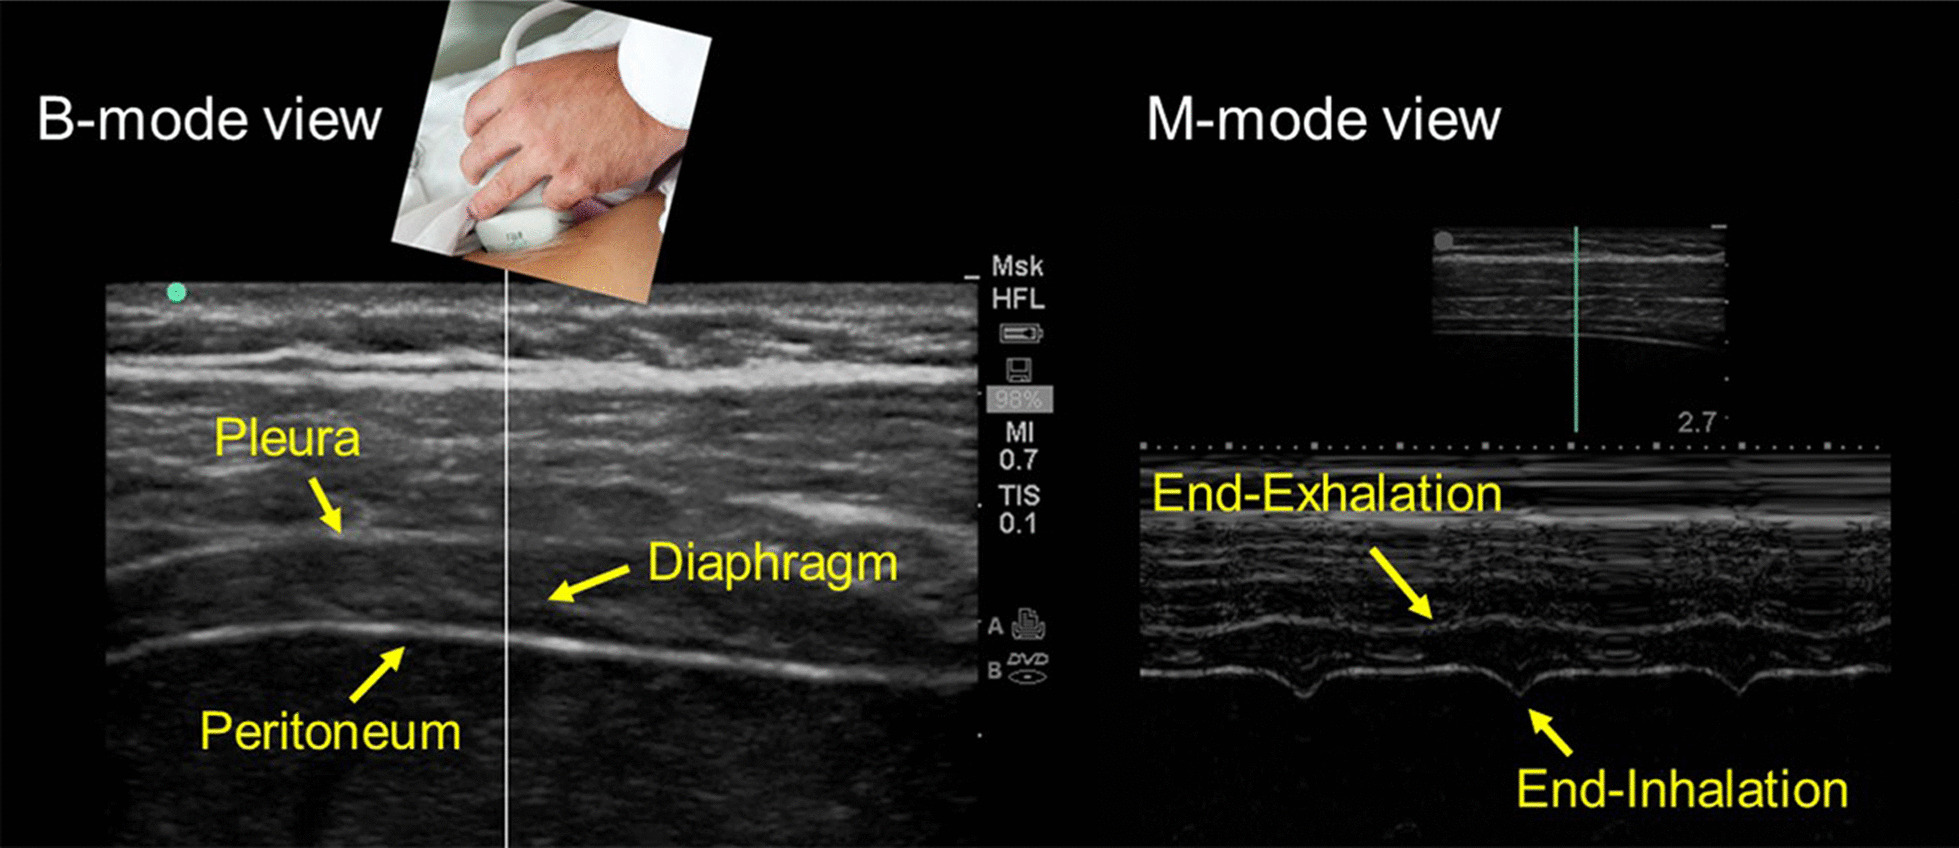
Ultrasound image of the zone of apposition of the diaphragm. In brightness-mode (B-mode; *left panel*), the diaphragm appears as a three-layer structure. In motion-mode (M-mode; *right panel*), the diaphragm is thinnest at end-exhalation and thickest at end-inhalation; stronger diaphragmatic inspiratory efforts are associated with greater tidal thickening of the diaphragm. Reproduced with permission from The American Association for Respiratory Care: Shaikh et al. Respir Care 2019;64:1600–2. The entirety of both images was obtained by Dr. Shaikh
